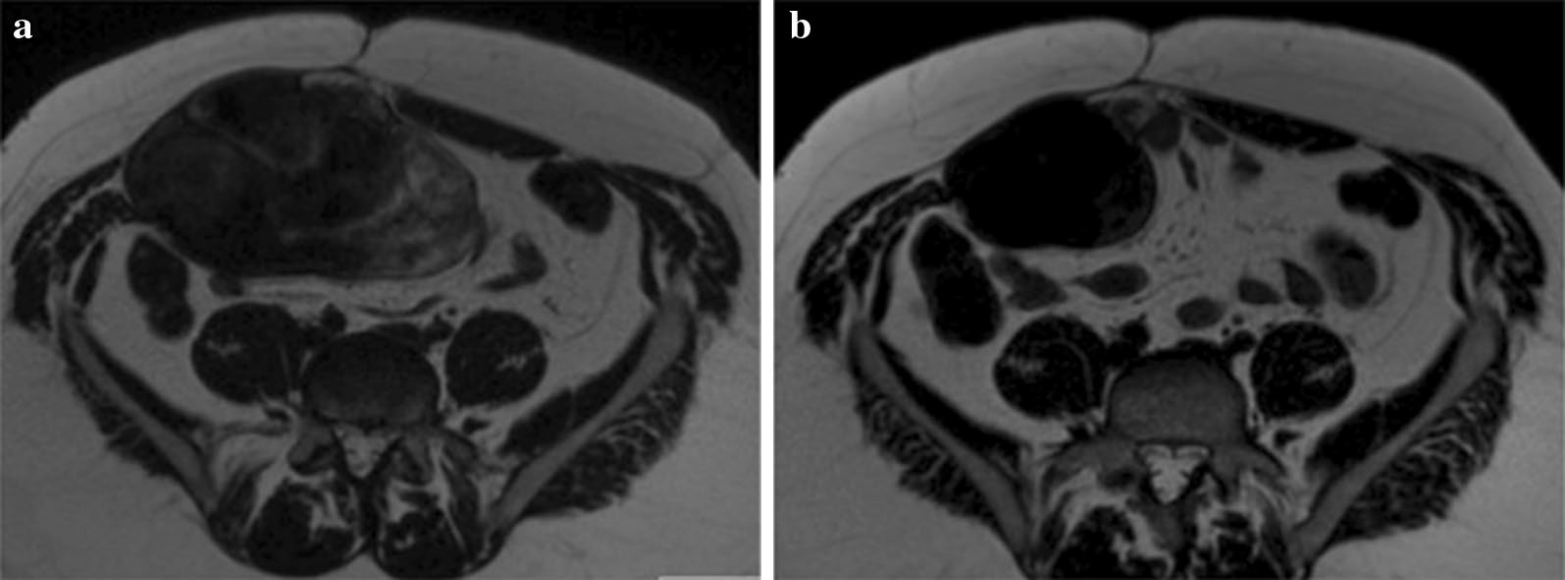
Axial T2 weighted MRI images showing right anterior abdominal wall fibromatosis in a 35-year old male (a) and the corresponding MRI after 7 months of treatment with tamoxifen (b) show decrease in size and T2 signal
